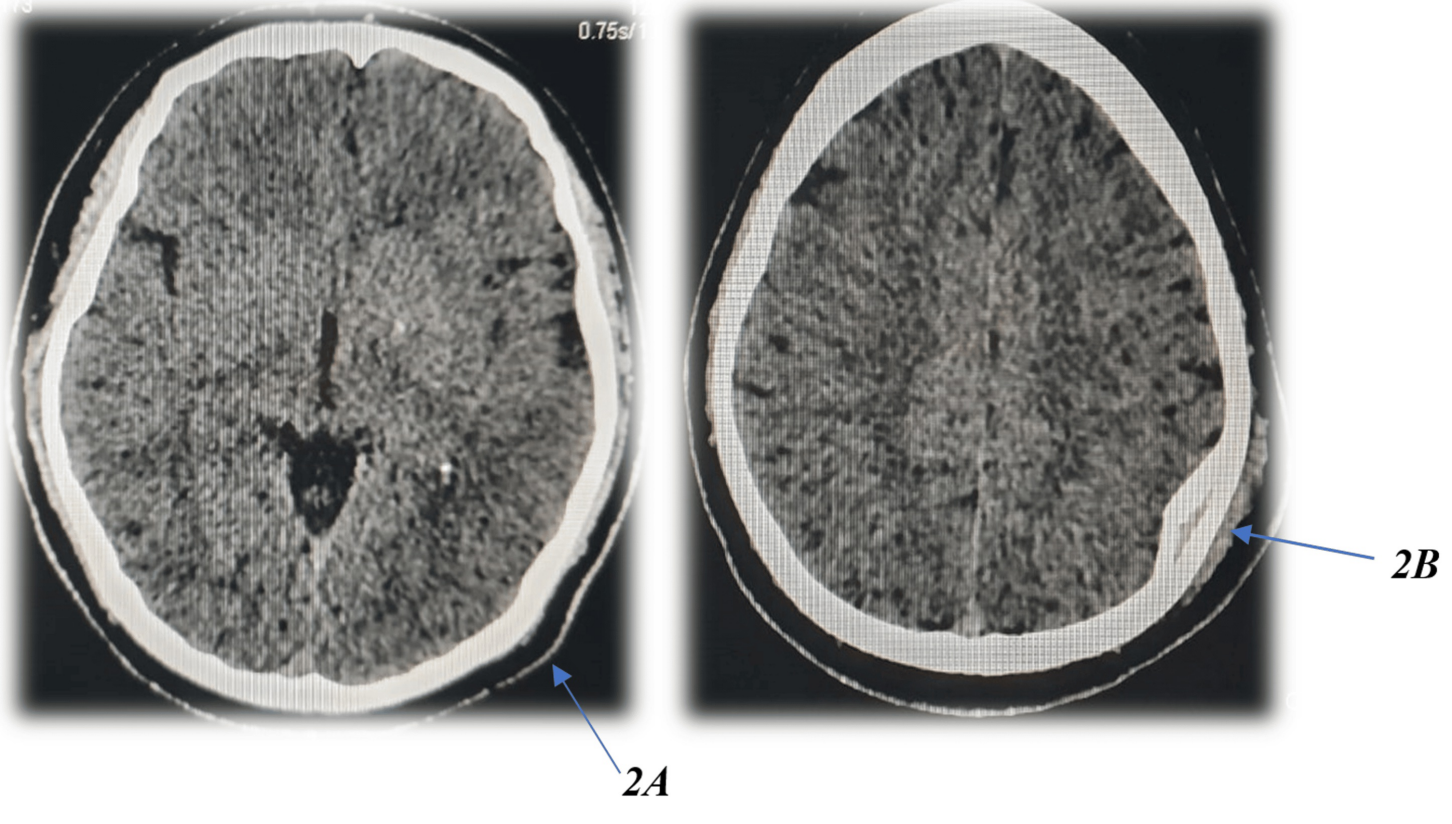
(A,B) Axial non-contrast cranial computed tomography (CT) scans (tissue window) demonstrating intact brain parenchyma, normal ventricular size, and absence of epidural, subdural, or intraparenchymal hemorrhage or contusion.
